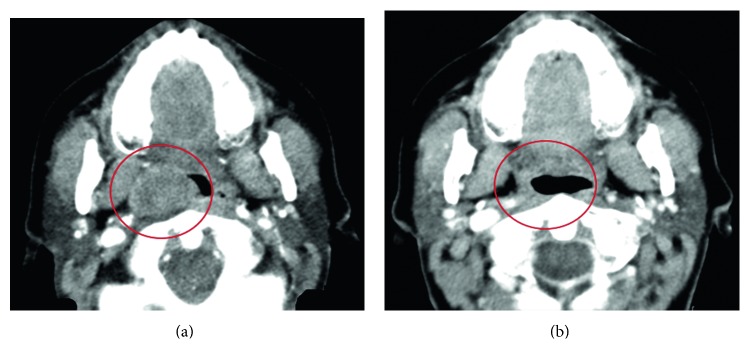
Changes in tumor size in patient 1. A CT scan of the neck, performed at the time of the second recurrence, showing an endophytic mass, with 3.1 × 2.2 cm, on the right oropharyngeal wall, with reduction of its caliber (a). Recent reevaluation showing sustained complete response to vemurafenib, 34 months after starting the treatment (b).
